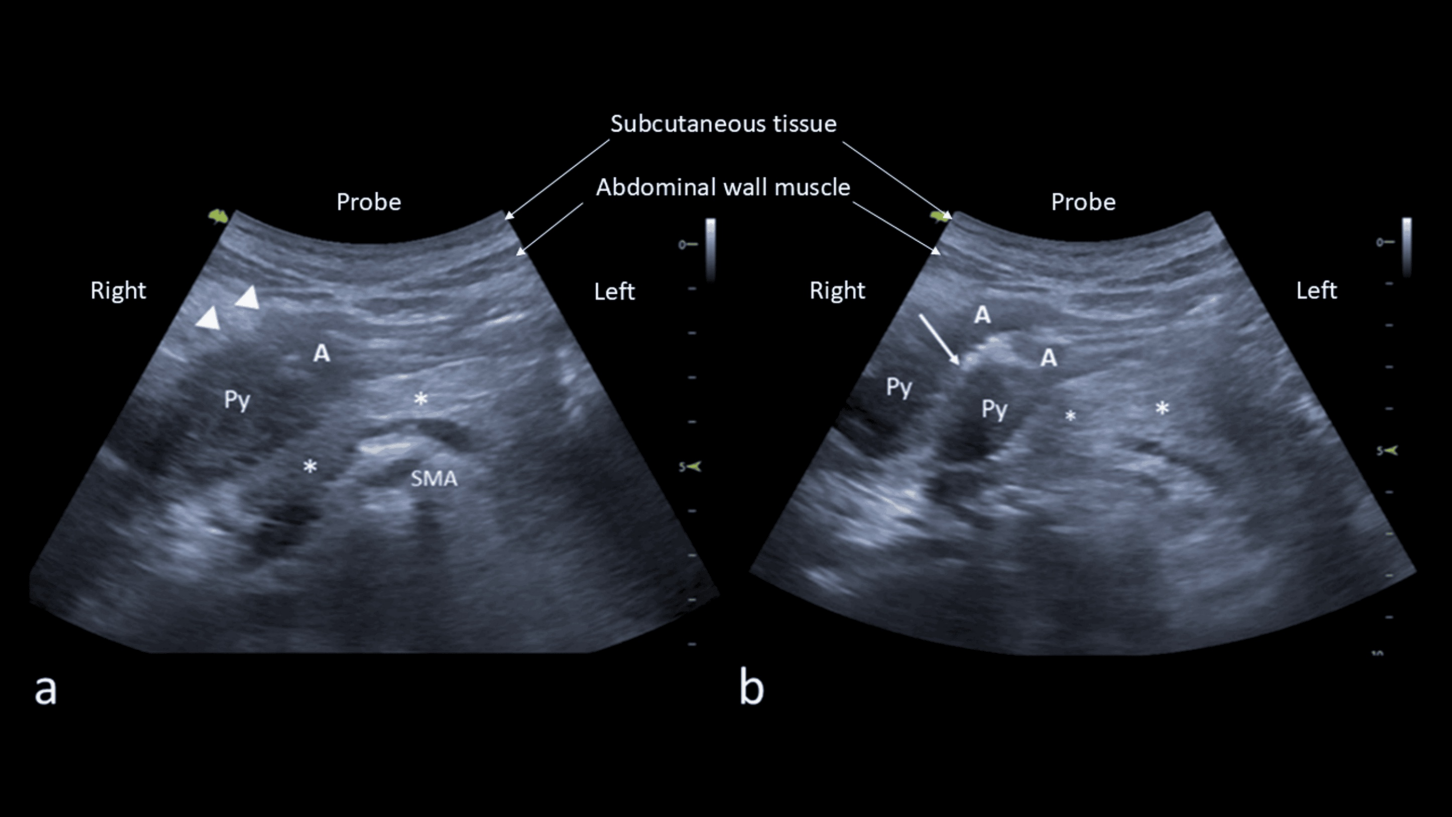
Transverse POCUS images (4.5 MHz) of the epigastrium; a) showing thickened gastric antrum (A) due to the tumor extending to the pylorus (Py) with outer border having an irregular margin indicated by arrowheads located anterior to the pancreas (*), and b) showing the tumor affecting the antrum and pylorus. POCUS: point-of-care ultrasound, A: antrum, Py: pylorus, SMA: superior mesenteric artery.
